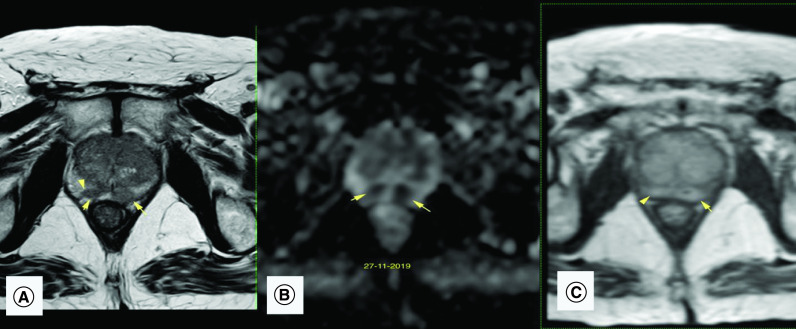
Multiparametric prostatic MRI after intravesical bacillus Calmette–Guérin therapy. (A) Axial T2-weighted image: a focal and ill-defined hypointense lesion in the right and left PZ (arrow) exhibits a bulging contour (arrowhead), suspicious of prostate cancer. (B) Apparent diffusion coefficient map: the PZ lesions (arrows) exhibit very low signal intensity in the apparent diffusion coefficient map. This is compatible with significant diffusion restriction in those areas. (C) Dynamic contrast enhancement shows moderate to significant early and diffuse enhancement mainly in the PZ. These findings are compatible with a PI-RADS score of 5. PZ: Peripheral zone.
